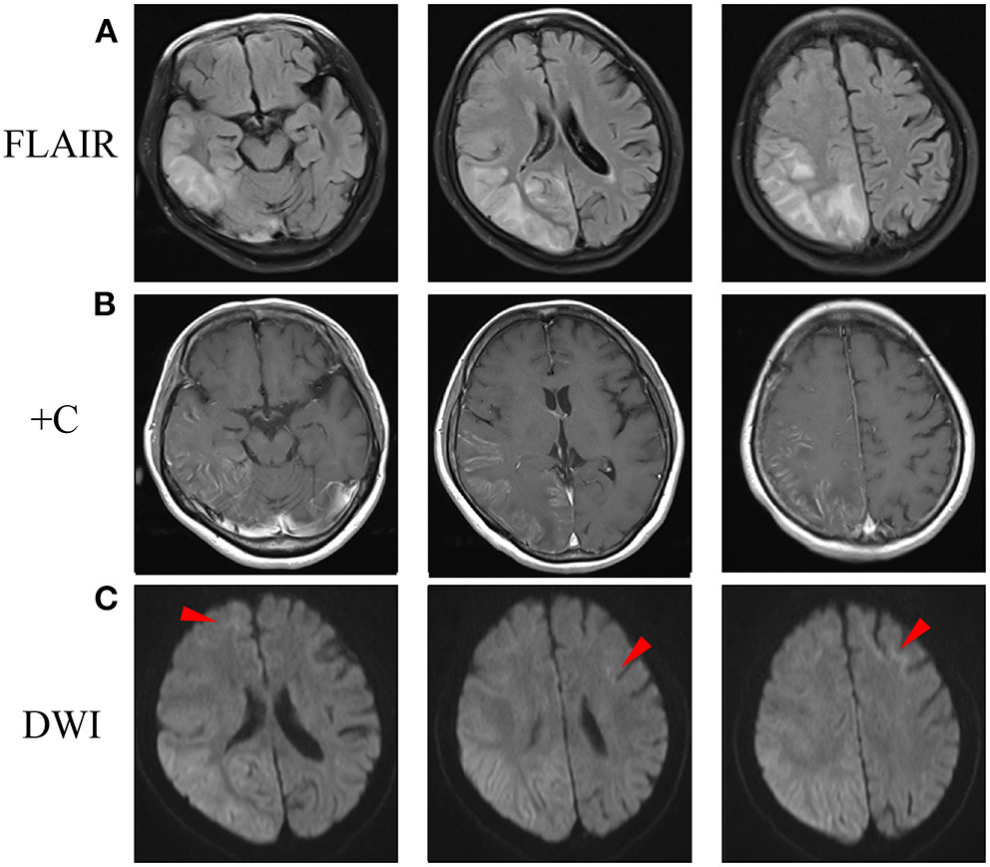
Brain MRI scan after admission. (A) FLAIR images showed abnormal hyperintensity along the cortex and partial subcortical area in the right occipito-parieto-temporal lobes and a part of frontal lobe. (B) Post-contrast MRI showed enhanced lesions along the surface of the cortex. (C) DWI showed hyperintensity in the right cerebral and corticomedullary junction of anterior frontal lobes (arrowheads). DWI, diffusion-weighted image; FLAIR, fluid-attenuated inversion recovery.
